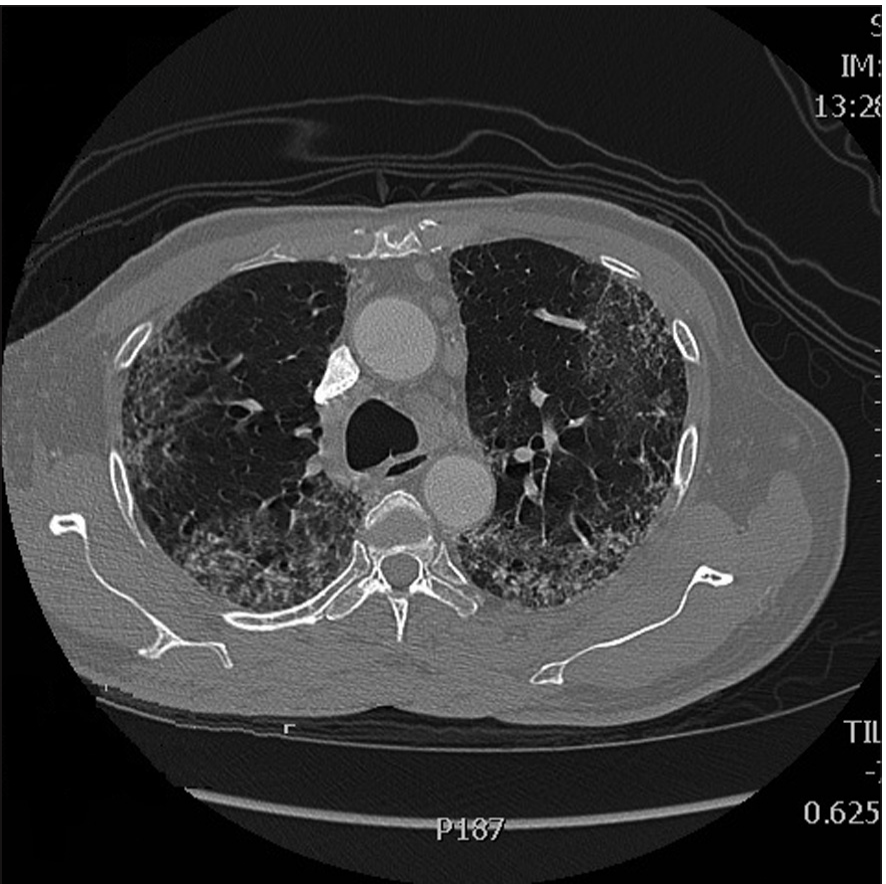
High resolution computed tomography scan 1
